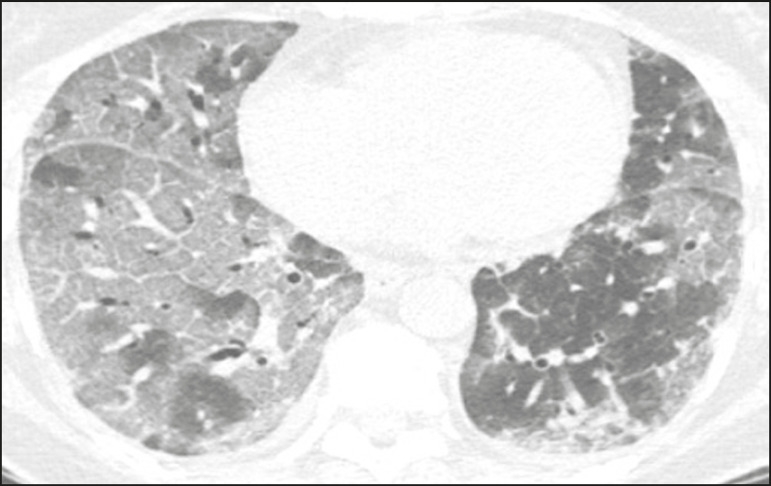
Chest CT scan of a 52-year-old male patient with a 6-day history of anosmia and dry cough who tested positive for SARS-CoV-2 on RT-PCR, showing a bilateral crazy-paving pattern, with ground-glass opacities and interlobular septal thickening.
